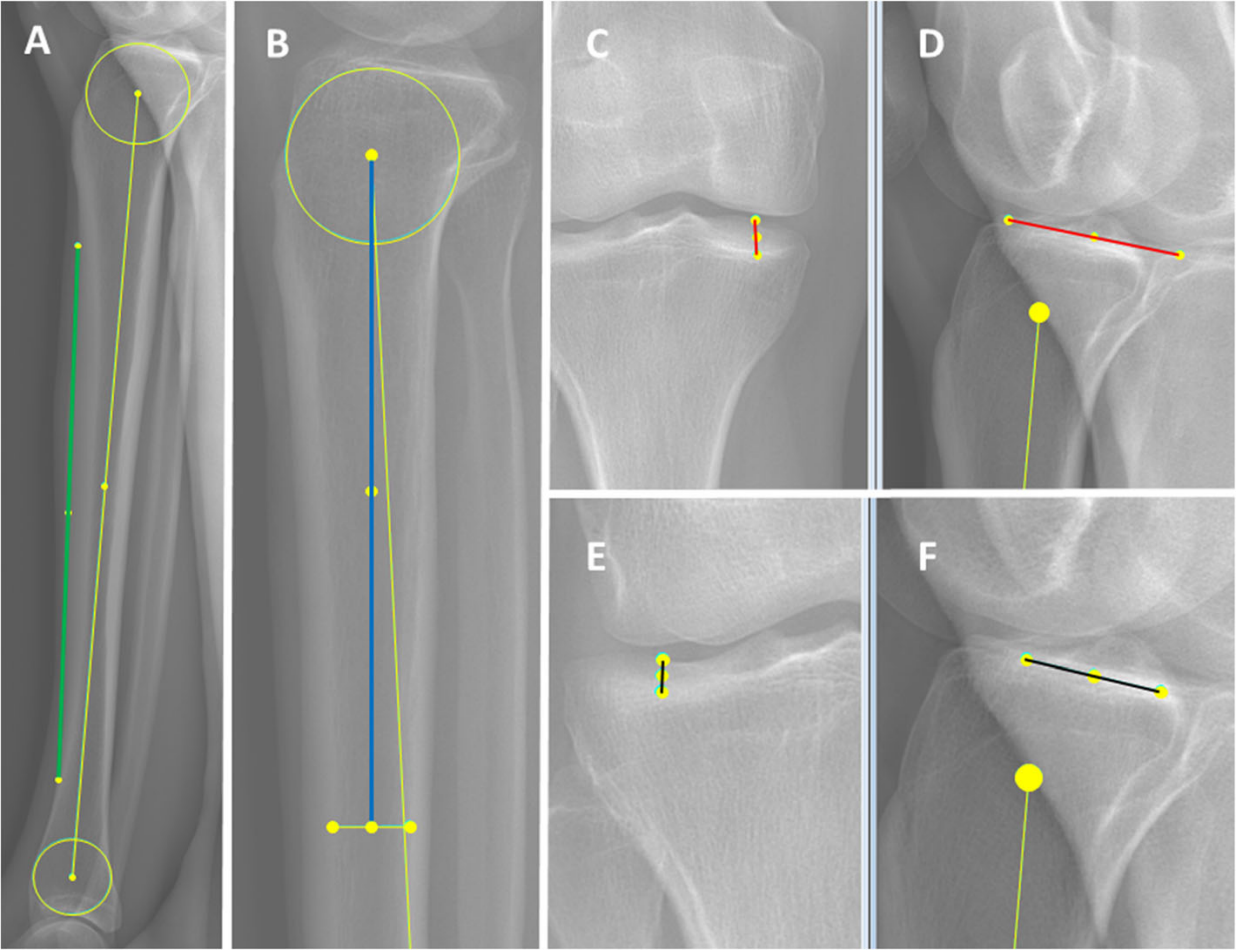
Depiction of the reference axes and slope measurements on EOS radiographs. (A–F) The yellow line is the TA on the sagittal image plane. (A, B, D, F) The green line is the ATC. (A) The blue line is the IMA. (B) The medial (C, D) and lateral (E, F) PTSs were measured on the sagittal image (D, F). The coronal image (C, E) was used mainly to identify the highest anterior point of the tibial plateau. The yellow dots are linked 3 dimensionally in the coronal and sagittal images
